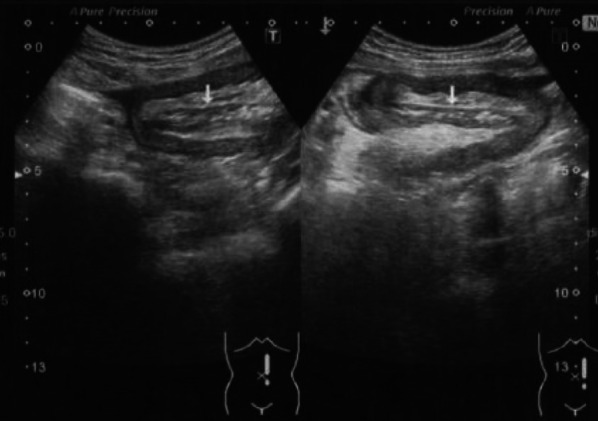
Abdominal ultrasonography. A small amount of ascites in the cysto-rectal fossa, a high echogenic mesentery, and small intestinal insertions in the intestinal tract of the left abdomen can be noted. Oral bowel dilatation was also observed
